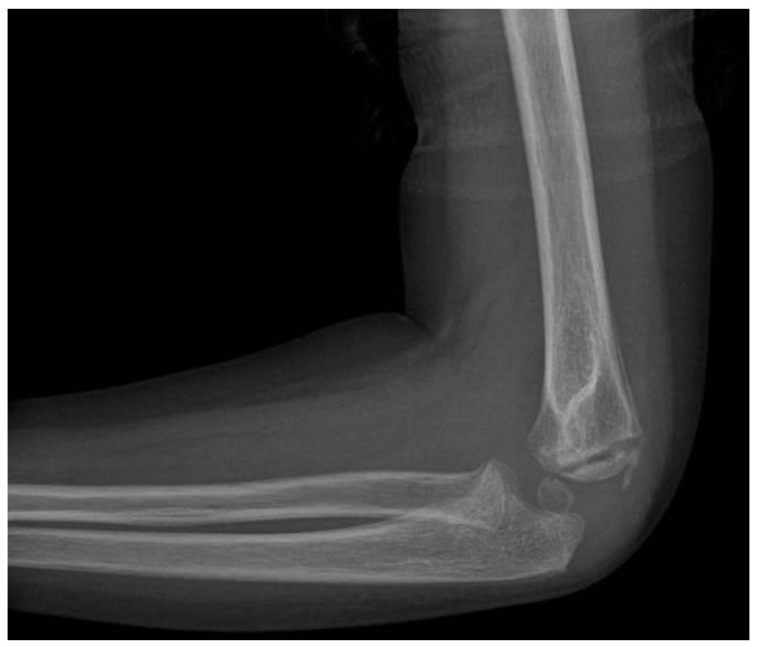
Postoperative X-ray after 30 days of immobilization (lateral view).
